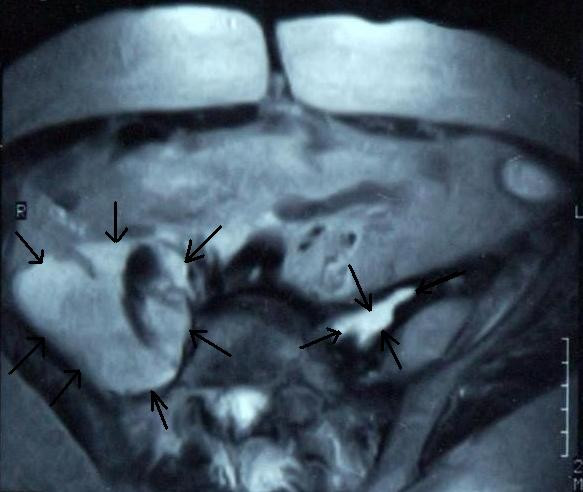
Abdominal MRI showing bilateral psoas muscle abscesses (arrows).
